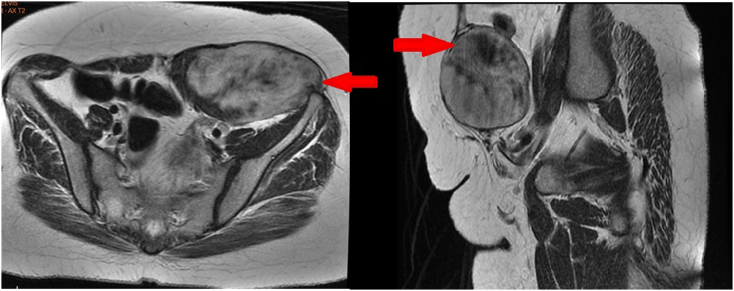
Abdominal MRI showing a mass located in the left abdominal wall reaching the left iliac crest measuring 11*6 cm with heterogeneous hyperintensity in T2-weighted.
